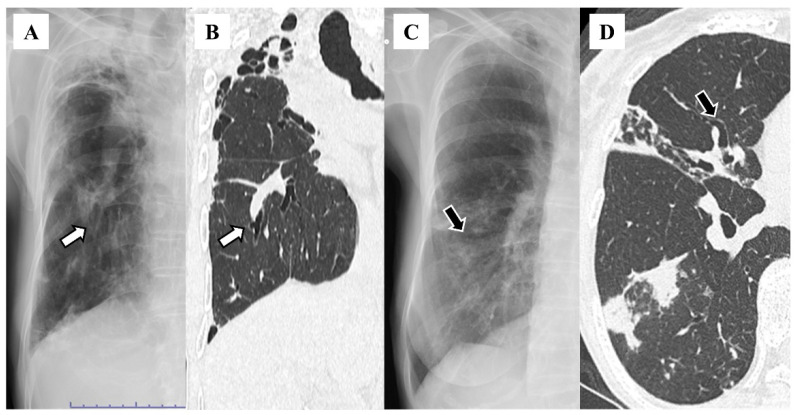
Tram-track appearance on chest X-ray (A) and high-resolution computed tomography (B). White arrows indicate the same lesions within the patient. Ring sign on chest X-ray (C) and high-resolution computed tomography (D). Black arrows indicate the same lesions within the patient.
